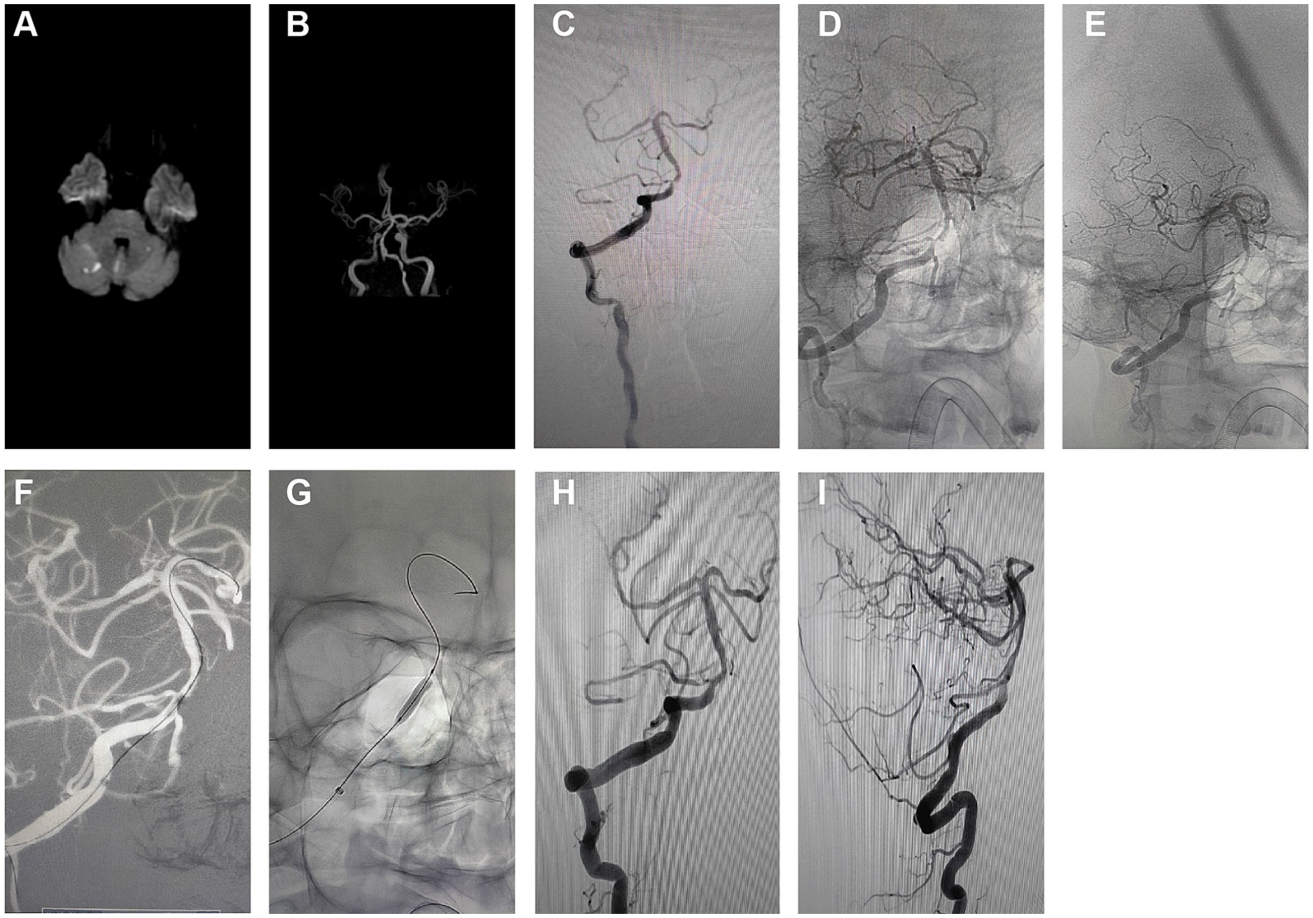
Short-term effects and perioperative safety analysis of PCB angioplasty. (A) Initial MRI results indicated multifocal high signals in the cerebellar hemispheres and vermis, suggesting acute infarctions. (B) Initial head MRA revealed significant stenosis to near-occlusion in the right vertebral artery V4 segment. (C) Pre-intervention angiography showed high-grade stenosis in the right vertebral artery V4 segment. (D) Pre-treatment anteroposterior angiographic images were used to evaluate the location of the stenosis. (E) Pre-treatment oblique angiographic images further clarified the morphology and extent of the stenosis. (F) Angiographic images during balloon expansion depicted the direct radiographic representation of vascular dilation. (G) Post-stent implantation angiography exhibited the stent placement and vascular morphology. (H) Post-treatment anteroposterior angiographic images demonstrated the restoration of blood flow in the stenosed area. (I) Post-treatment lateral angiographic images provided an alternative view of the vascular patency.
